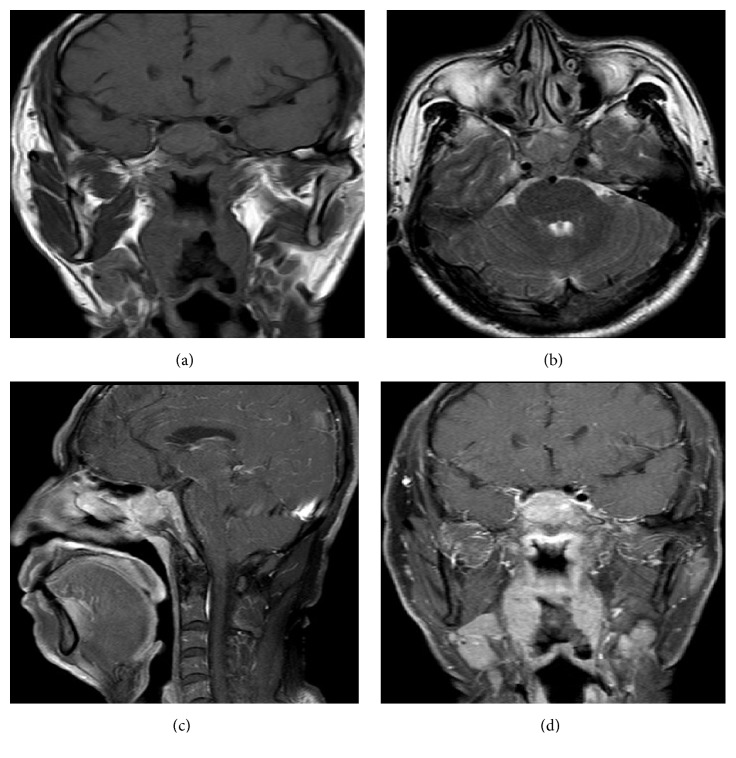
Sagittal T1-weighted magnetic resonance image (MRI) and coronary T2-weighted MRI revealed a mass occupying the sphenoidal sinus (a,b). Gadolinium-enhanced MRI demonstrated the neoplasm with homogenous soft-tissue lesion occupying the sphenoidal sinus and destroying the clivus (c,d).
